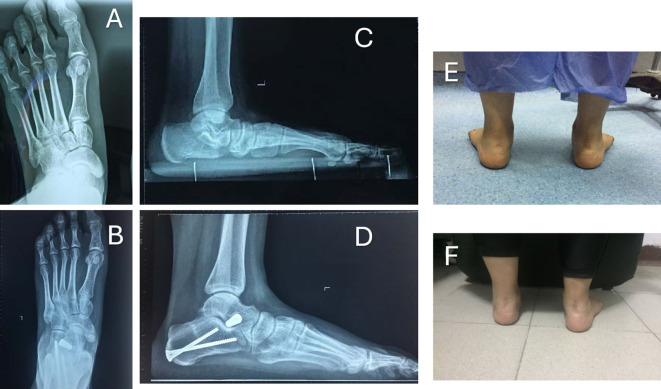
Pre- and post-operative X-rays and clinical photographs of 20-year-old female underwent left foot MDCO + STA. A: Preoperative A-P weight-bearing X-ray. B: Postoperative A-P weight-bearing X-ray. C: Preoperative lateral weight-bearing X-ray. D: Preoperative lateral weight-bearing X-ray at the last follow-up. E: Preoperative clinical photo showing the left foot hindfoot valgus. F: Postoperative clinical photo with evidence left foot hindfoot valgus correction at the last follow-up.
